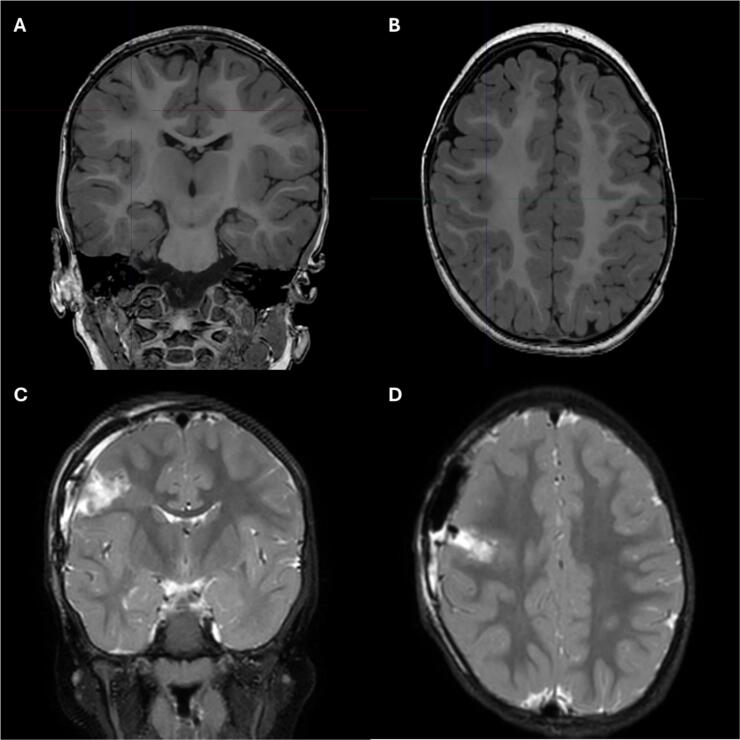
Magnetic resonance imaging (MRi): Coronal (A) and axial (B) T1-weighted 3 T MRI scans showing the dysplasia in the right central region, involving the central sulcus. Postoperative T2-weighted MRI confirmed resection of the dysplasia (C and D).
